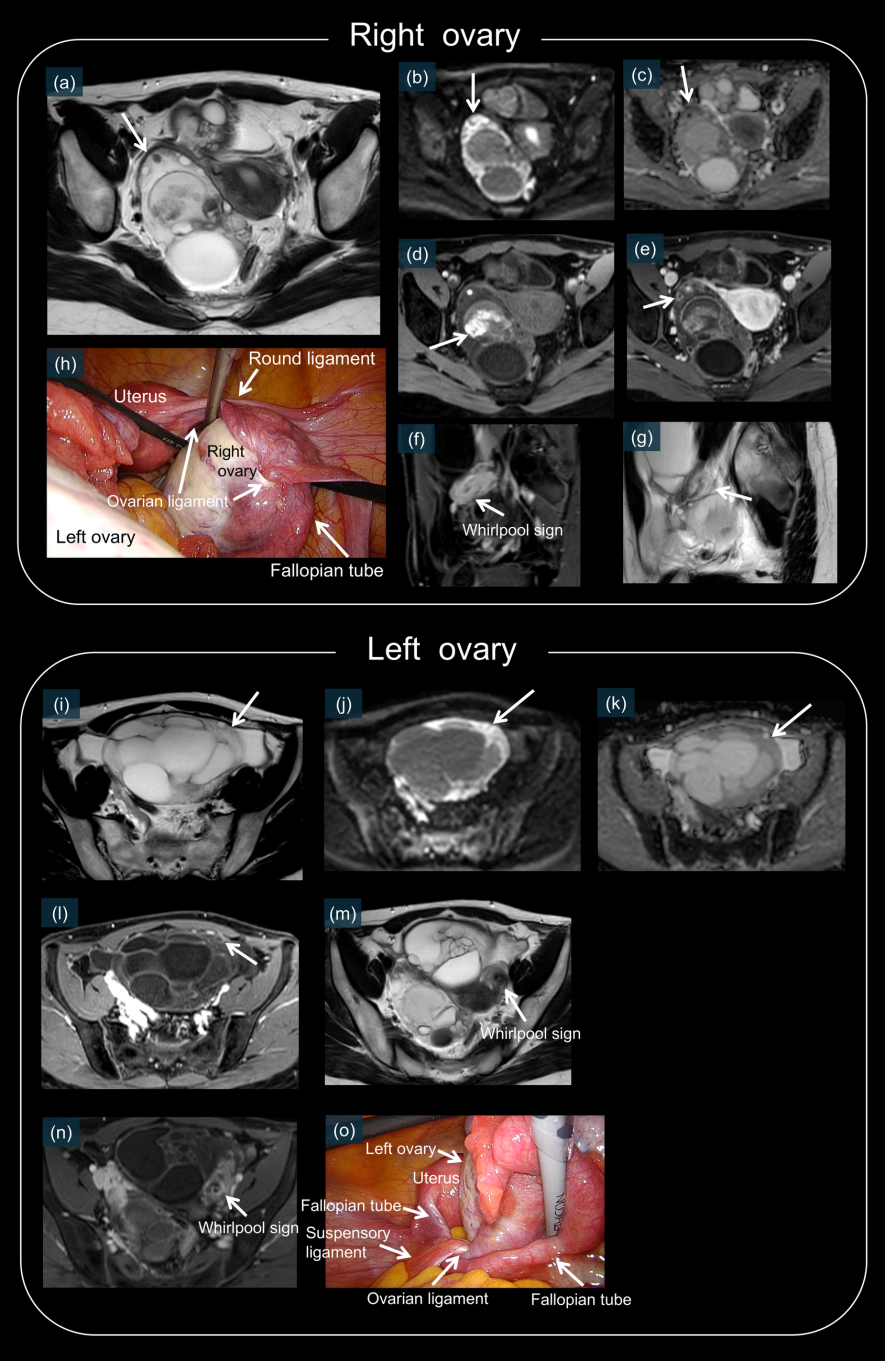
Both ovaries of a 27-year-old female patient are markedly enlarged, showing > 20 relatively large cysts, suggesting polycystic ovary syndrome. Partial hemorrhage was observed within a cyst in the right ovary on fat-suppressed T1WI (d, white arrow). Massive ovarian edema is partially observed in both ovaries, appearing as high signal intensity on T2WI reflecting edema (a and i, white arrows). These areas show diffusion restriction on DWI/ADC (b, c, j, and k, white arrows). Hemorrhagic infarction may be associated with a high signal intensity on DWI. On postcontrast sequences, enhancement was observed in the periphery of the ovaries and follicles (e and l, white arrows). Twisted pedicles are seen bilaterally (f, g, m, and n, white arrows), with whirlpool signs visible (f, m and n). h and o are laparoscopic images revealing that both ovaries are enlarged and twisted (right, 810°; left, 720°)
